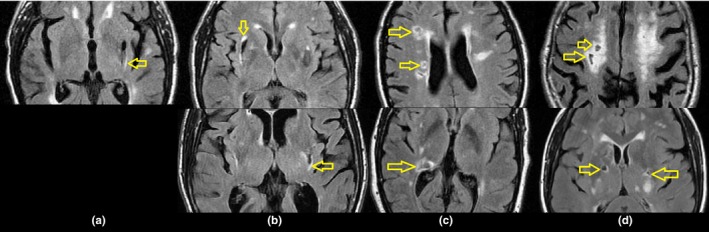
Cavitated white matter hyperintensities [cavitated white matter hyperintensities (WMH)]: in contact with lacunes but without overlap (a), partially overlapping with lacunes (b), surrounding (or completely overlapping with) cavities “in formation” (or possibly lacunes affected by partial volume effects due to Magnetic Resonance Images (MRI) slice thickness) (c), and totally overlapping with lacunes (d). The characteristic locations for each ‘class’ are shown
